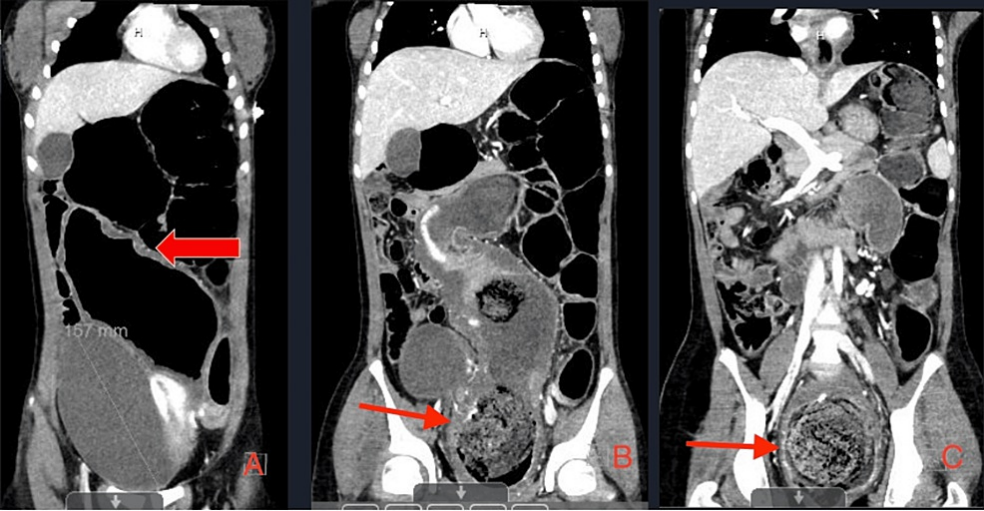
CT of the abdomen and pelvis showing diffusely dilated loops of bowel with evidence of a fecaloma. Image A demonstrates areas of dilated loops of the bowel with the red arrow pointing to a thickened section of the intestinal wall, which is suggestive of stercoral colitis. Image B demonstrates dilated loops of the bowel with the red arrow pointing to a large, obstructing fecaloma. Image C further demonstrates dilated loops of the bowel with the red arrow pointing to the obstructing fecaloma in greater detail.
